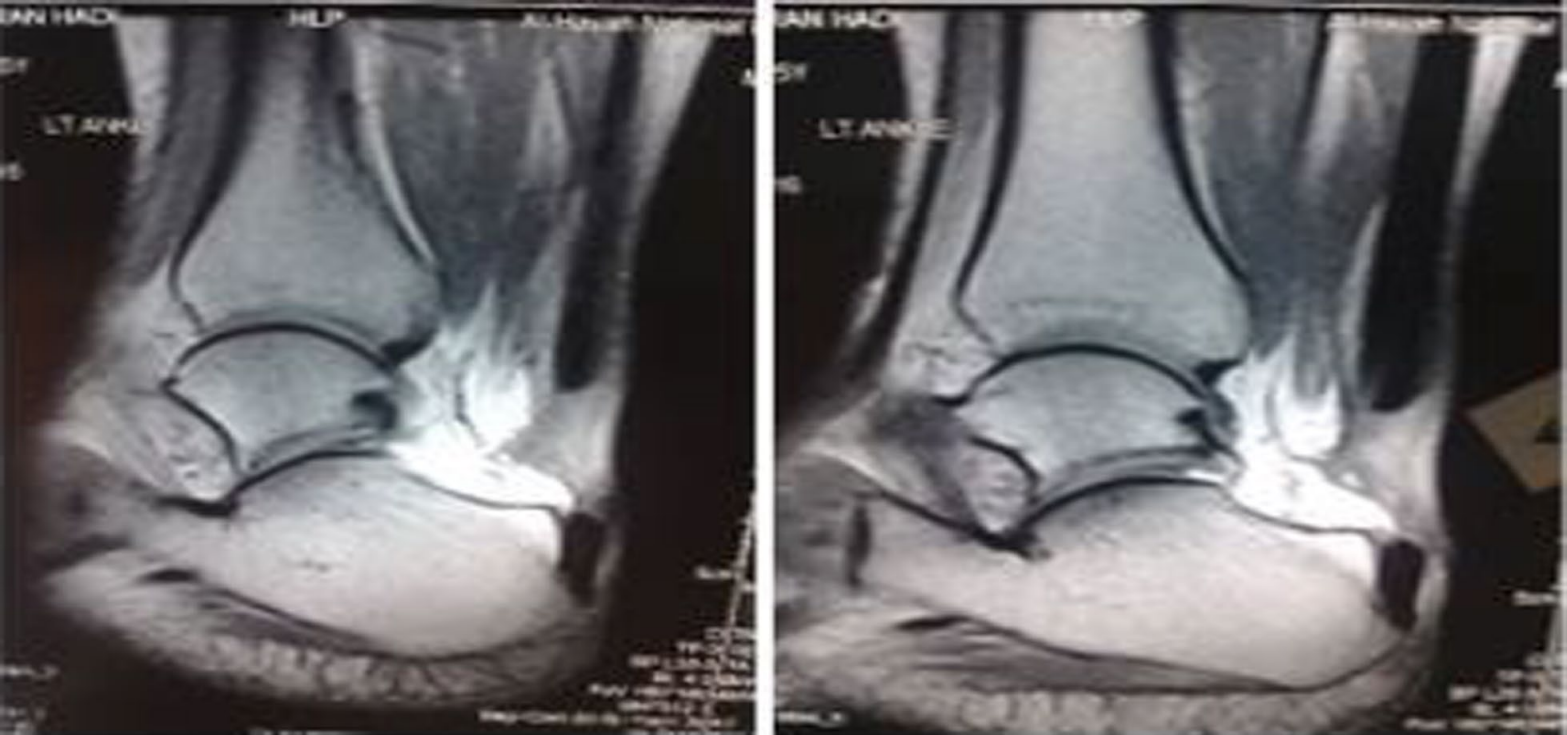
MRI image showing a gap greater than 2 cm in the torn Achilles tendon
